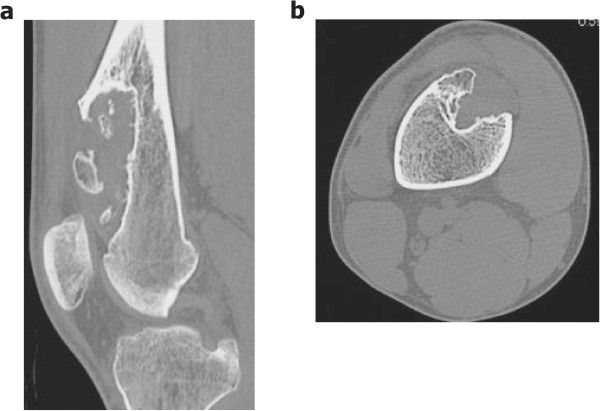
Preoperative CT. (a) Sagittal and (b) axial scans, showing an extraskeletal mass with focal calcification on the anterior surface of the right distal femur.
